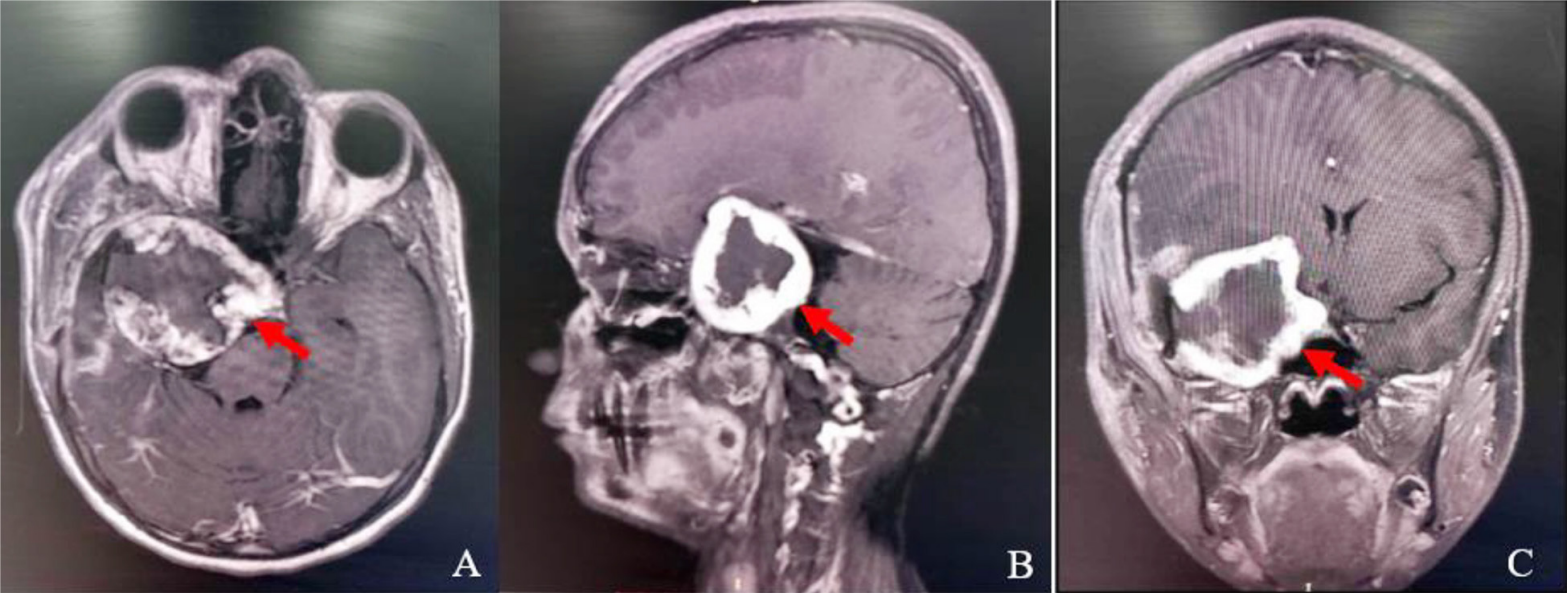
A: MRI brain with IV contrast showing peripherally contrast enhancing right extra-axial space occupying lesion (represented by red arrow). B: Sagittal view. C: Coronal view.
